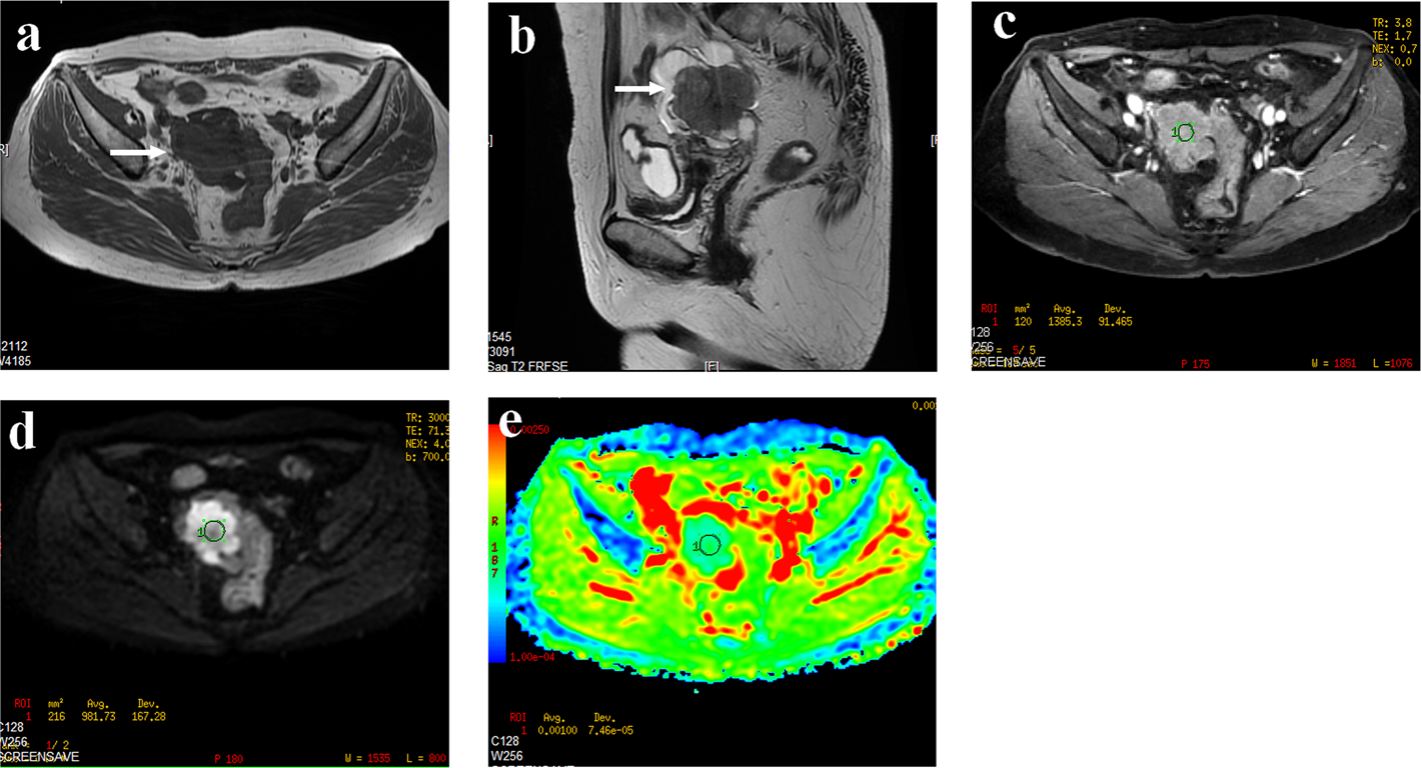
A 59-year-old female patient with serous cystadenocarcinoma. (a) Axial T_1_WI revealed a lobulated solid mass with isointensity in the right adnexal region (arrow). (b) On sagittal T2WI, the solid tumor displayed homogeneous isointensity. (c) On contrast-enhanced fat-suppressed T_1_WI, the lesion showed mild enhancement. (d) On DWI-MRI (*b* = 700 s/mm^2^), the lesion appeared as hyperintensity. (e) ADC map demonstrates homogeneous isointensity, with an ADC value of 1.00 × 10^–3^ mm^2^/s.
